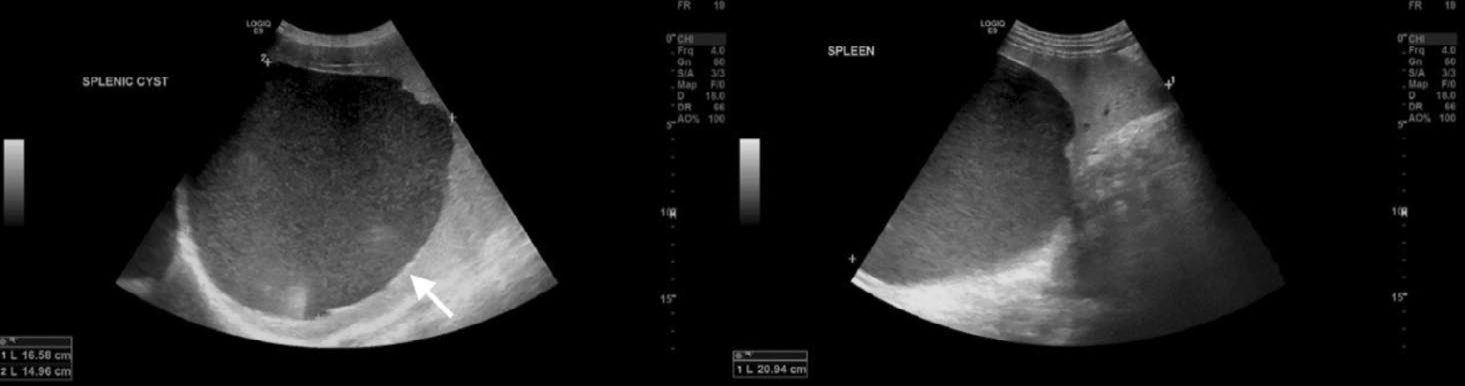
Gray scale ultrasound of the abdomen showing large well defined hypoechoic lesion occupying the upper pole of the spleen, showing internal echoes with posterior acoustic enhancement, few linear hyperechoic discontinues wall lesions suspicious for calcification (a, arrow). No internal vascularity on color Doppler, no soft tissue or fat component.
